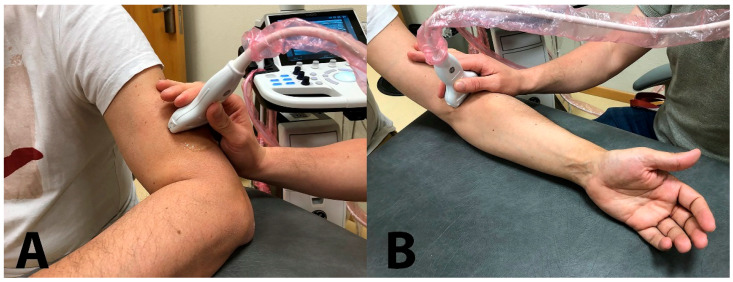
Ultrasound probe location for evaluation of the cross-sectional area of the radial nerve at the spiral groove (A) and the antecubital fossa (B).
